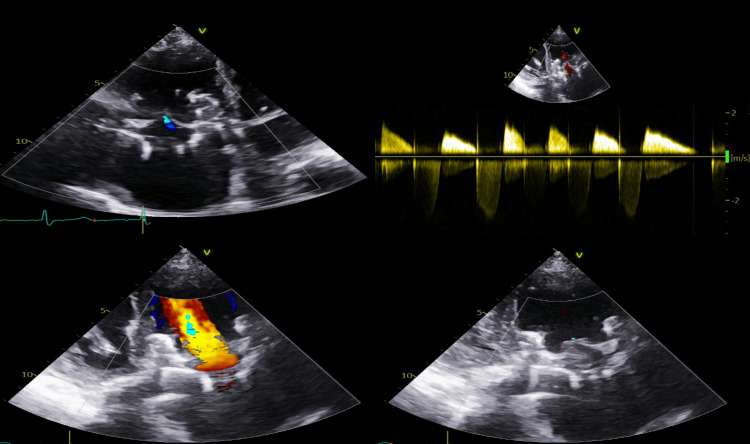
Echocardiogram images at follow-up showing a well-functioning transcatheter tricuspid valve bioprosthesis
